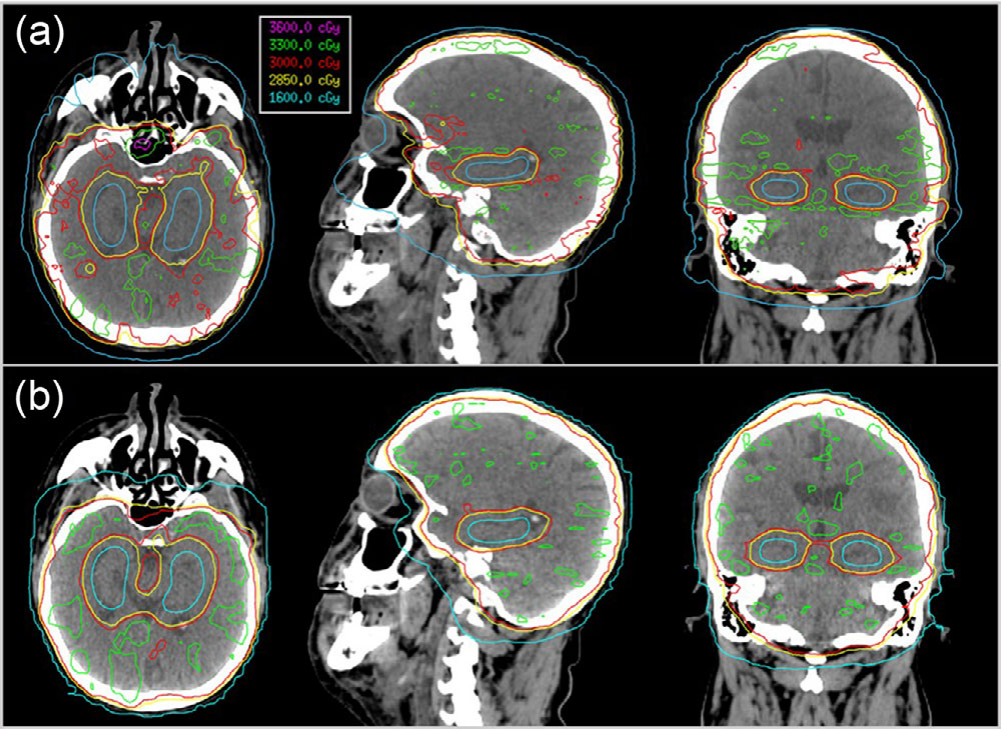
Comparison of achieved dose distributions for hippocampal avoidance (HA) whole brain radiotherapy (WBRT) in a representative patient plan. (a) MRI‐guided radiotherapy (MRgRT) with co‐planar fixed‐field IMRT. (b) Clinical plan with noncoplanar volumetrically modulated arc therapy (VMAT) on a conventional c‐arm linear accelerator
